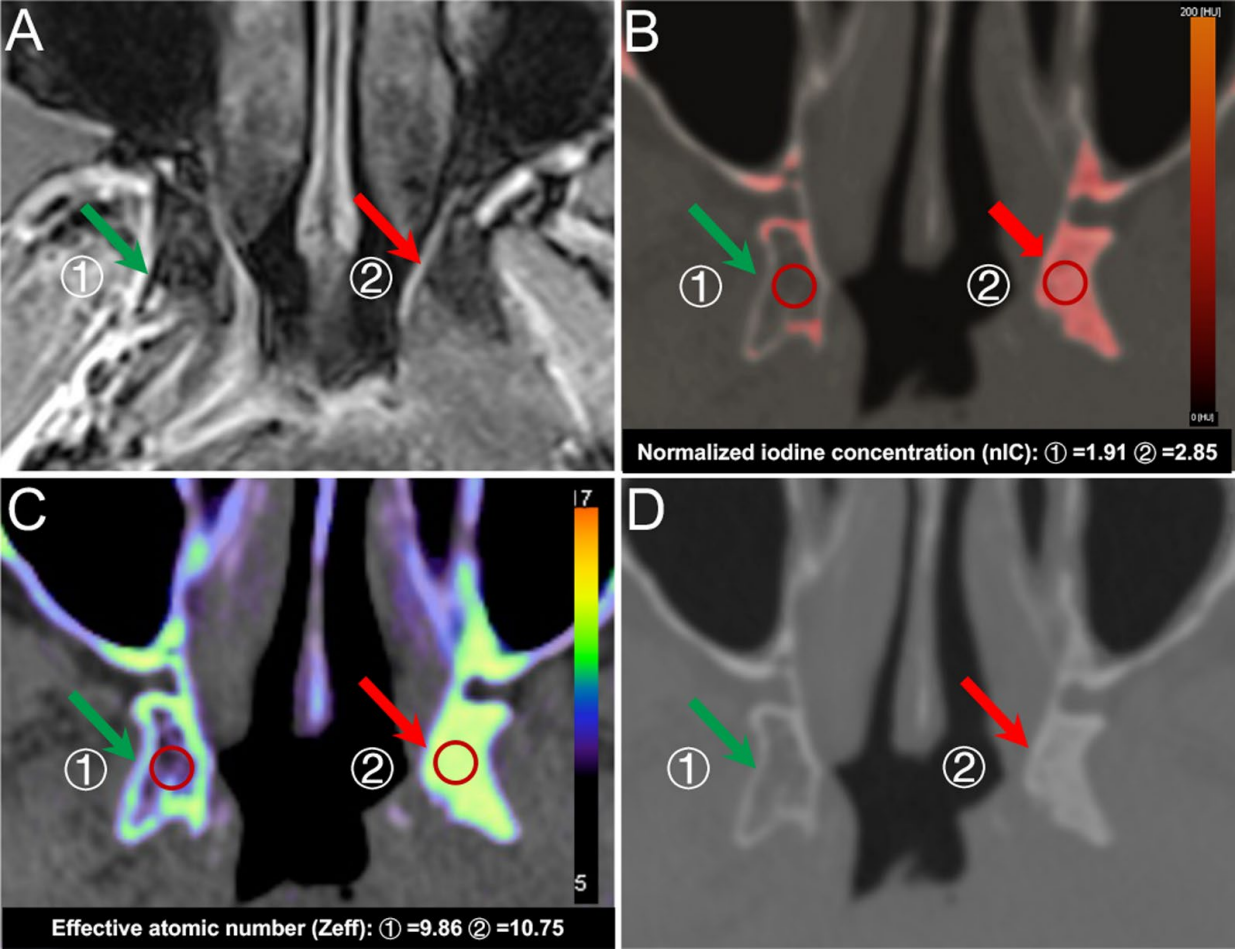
False-negative findings for skull base invasions by MRI in a 43-year-old man with NPC. A The axial MRI images (fat-suppressed T1-weighted image after gadolinium administration) do not show obvious abnormal contrast enhancement at the left pterygoid process (red arrow). The DECT images (B, IO image; C, Zeff image) directly show marked bone invasions with higher iodine concentrations than right pterygoid process (green arrow). D The SECT images (bone window) confirm the invasions with sclerosis at the left pterygoid process like the DECT images
